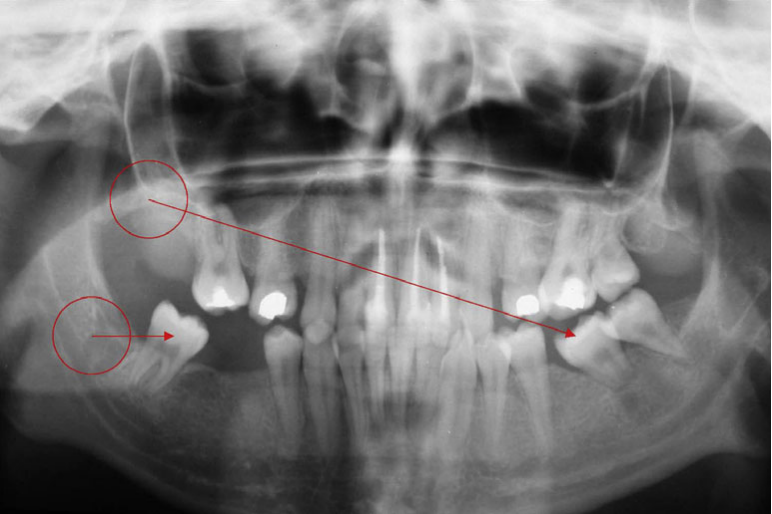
X-ray of the upper and lower jaw four months after surgery. Teeth 18 and 48 were extracted carefully and transplanted into position 36 and 47, respectively (marked by circles and arrows).
